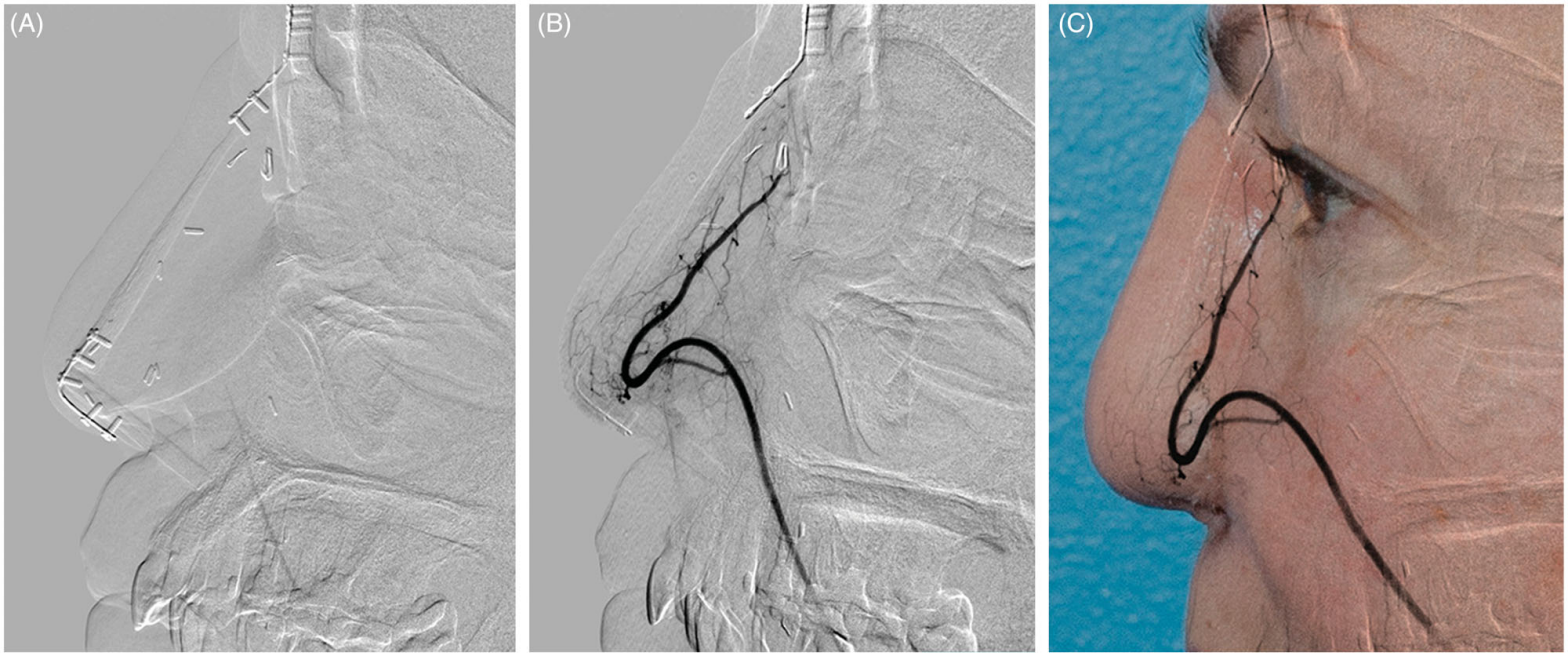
CT angiography of “neo-nose” vascularization. On the left side, picture shows nose just moment before contrast was injected into the pedicle (A, B). On the right side, CT angiography shows transparency to the actual patient face (C).
